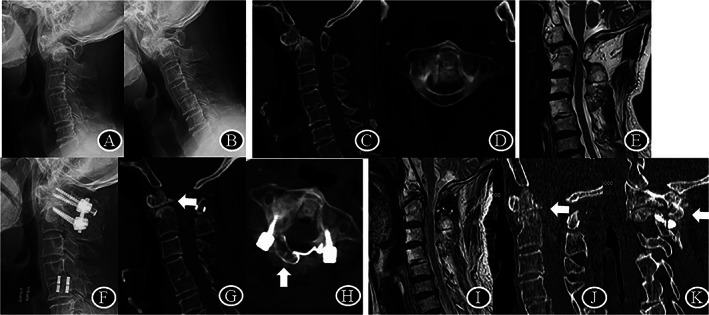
The imaging of patient no. 4, male, who suffered from an old and displaced odontoid fracture. (A–D), Preoperation SAC of 5 mm; (E), Preoperative midsagittal T2‐weighted MRI demonstrating the spinal cord was compressed by the dens and posterior ring of C1; (F–H), Postoperative CT scan, demonstrating full reduction of dens fracture and SAC of 12 mm immediately post‐operation; (I), Postoperative T2‐weighted MRI, demonstrating full decompression at 25 months after laminoplasty; (J, K), Sagittal CT scan, demonstrating successful dens fracture healing and interlaminar fusion between C1‐C2
